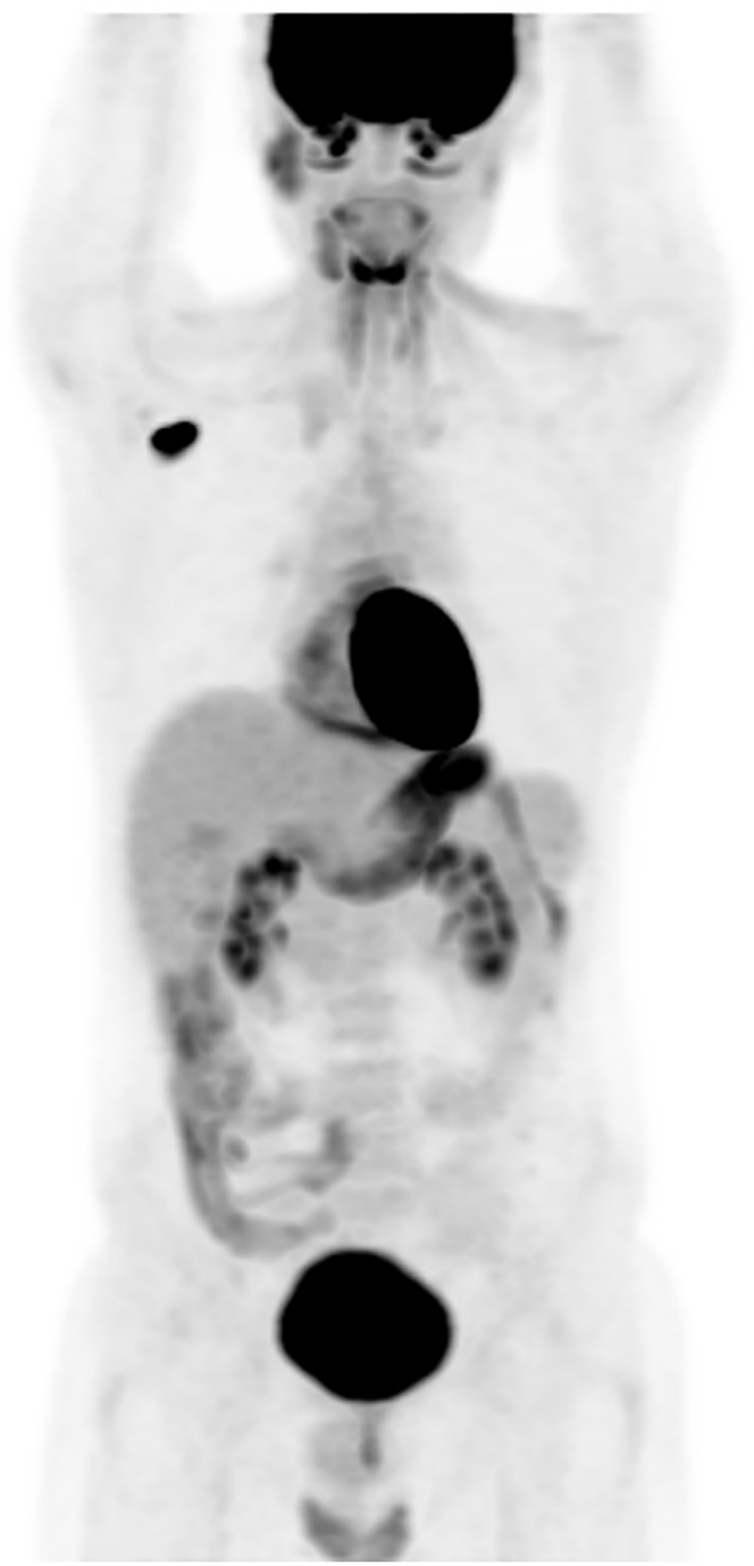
(PET-CT) scan: an isolated hypermetabolic focus in right enlarged axillary lymph node with SUV max of 13.4.
